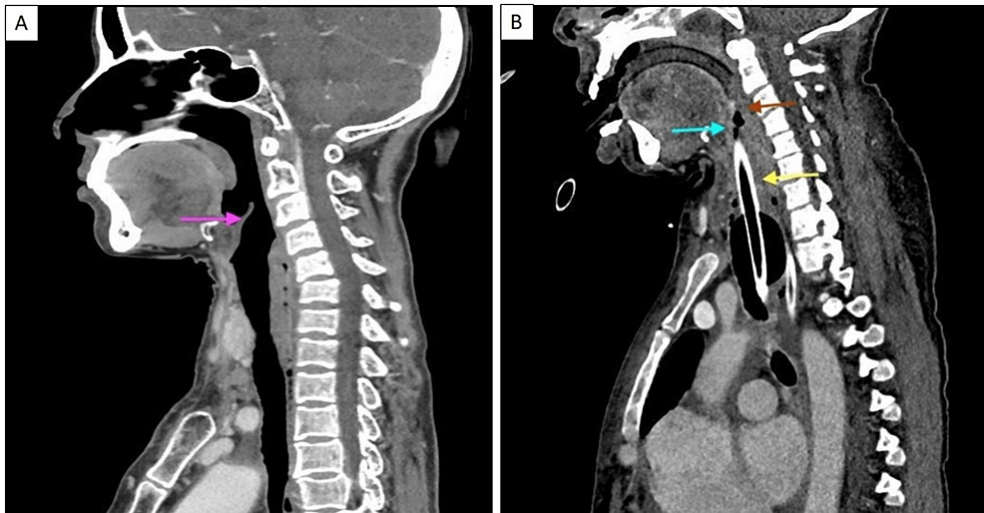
Sagittal CECT image of the face and neck of a normal patient (A) compared to our patient (B) Image A shows a sagittal reformatted CECT image of the face and neck showing normal oropharynx and larynx/hypopharynx with clearly delineated laryngeal cartilage/epiglottis (pink arrow), while image B shows sagittal reformatted CECT image of the face and neck of the patient showing diffuse edema in the oropharynx (red arrow) and larynx/hypopharynx (yellow arrow) causing airway compromise. Laryngeal cartilages/epiglottis (blue arrow) could not be well delineated. The endotracheal tube is in situ. CECT: Contrast-enhanced computed tomography.
